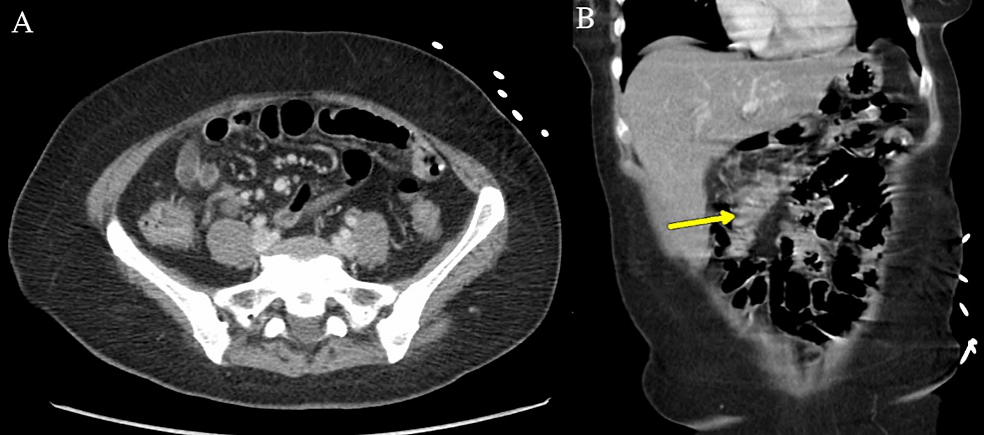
(A) Axial abdominal CT with mild transverse colonic wall thickening suspicious for colitis. (B) Coronal abdominal CT with mild ascending colonic wall thickening.
